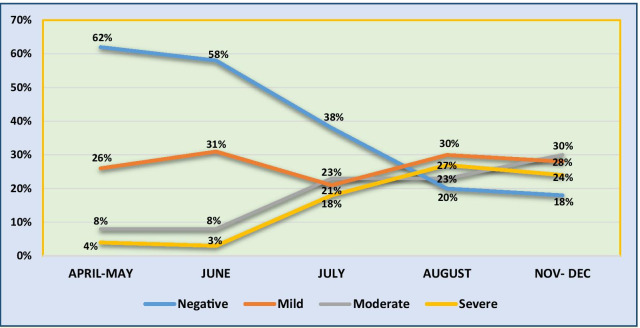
Trend of severity in eastern Indian population. *X*-axis—period in months. *Y*-axis—patient population. Mild, moderate and severe categories were divided on the basis of CT severity score as 1–5, 6–15 and 16–25, respectively
